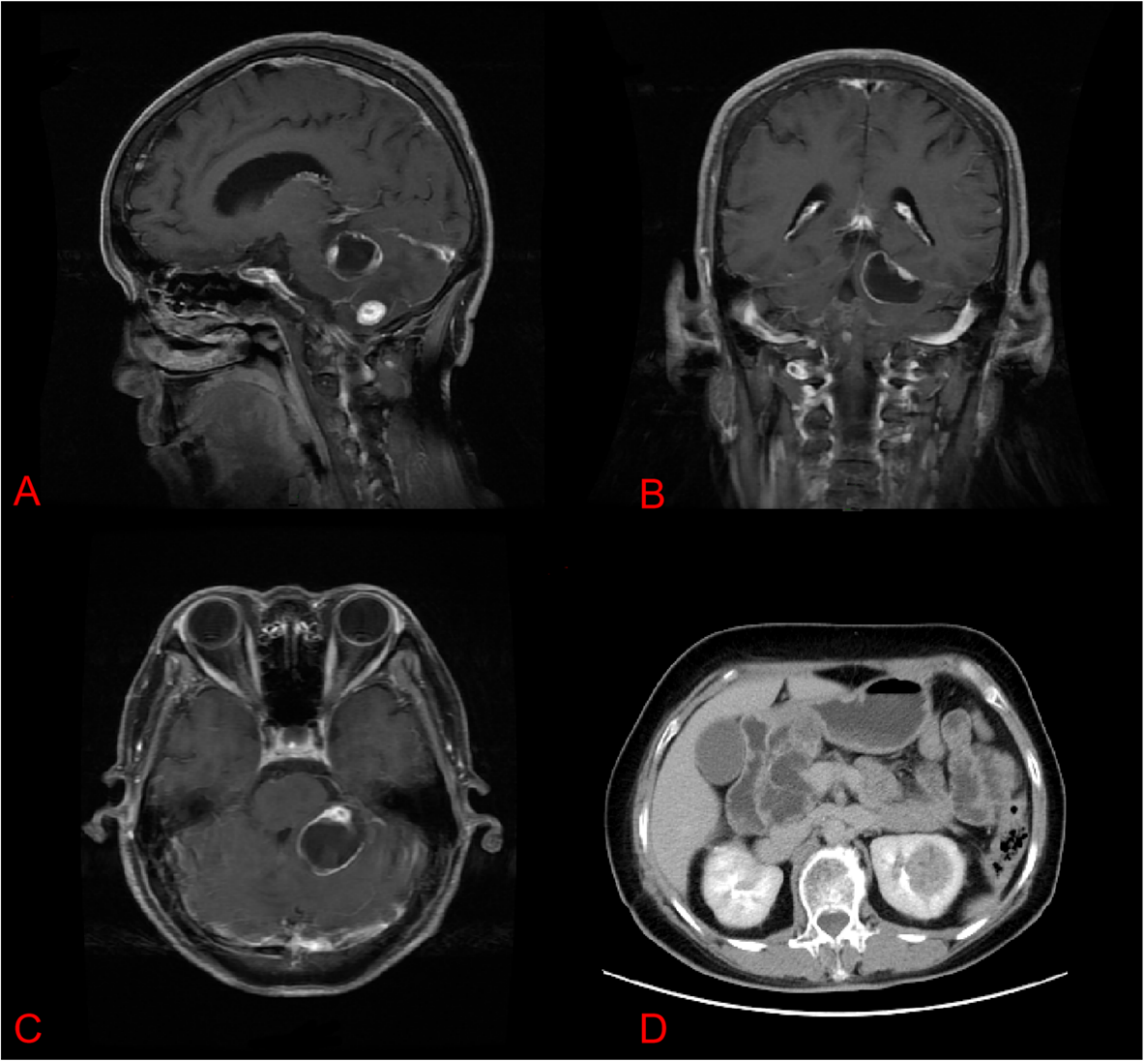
a–c Contrast-enhanced magnetic resonance imaging (MRI) of the cerebellum hemangioblastomas in the 58-year-old female patient. d. Abdominal computed tomography (CT) scan with contrast demonstrated renal cell cancer and multiple renal and pancreatic cysts in the same patient
